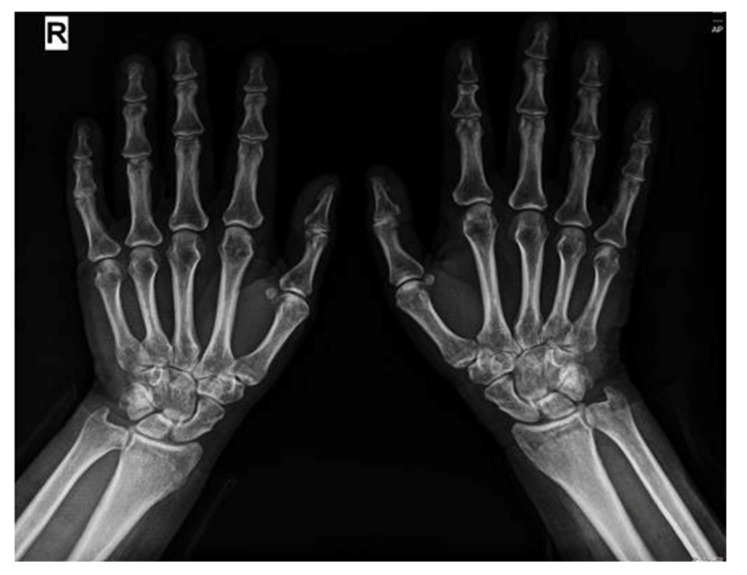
Hand X-ray showing non-erosive changes.
